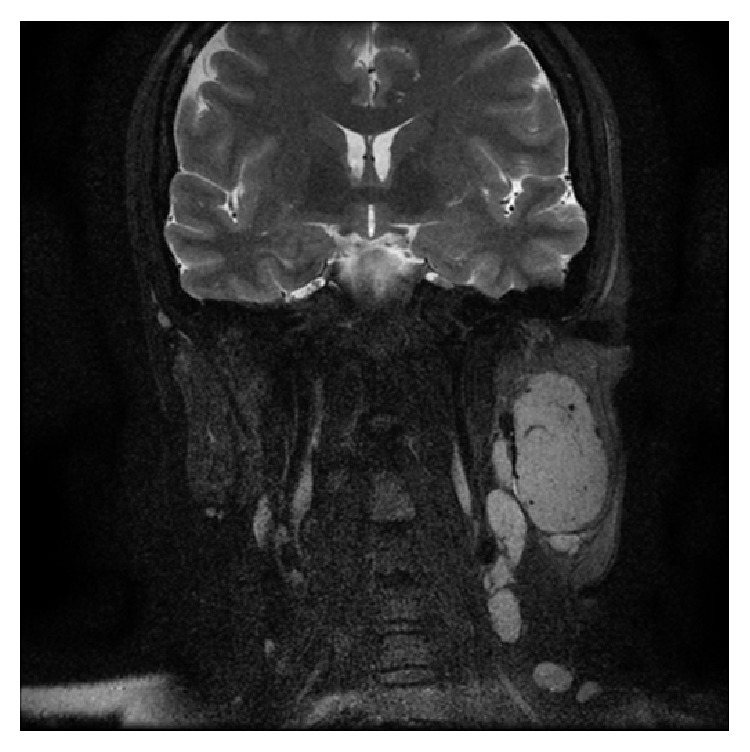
MRI head and neck coronal cut, after contrast, T2: a well-defined, T2 intermediate SI solid lesion in the left superficial parotid lobe. There is diffuse intense enhancement. Multiple enlarged lymph nodes in the left periparotid region.
